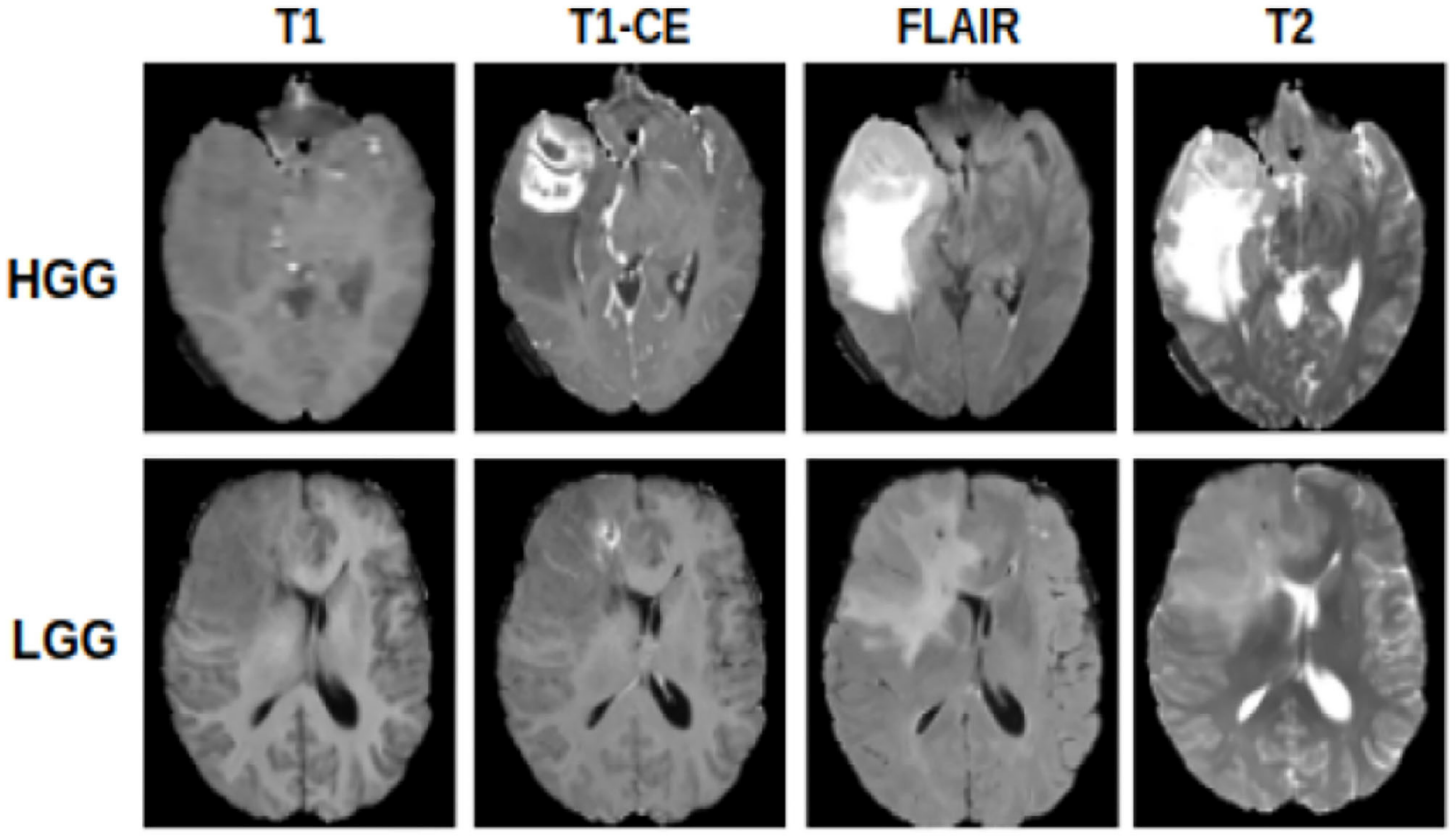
Examples of MRI slices of LGG and HGG samples. Each sample has four MR modalities, i.e., T1-weighted MRI, T1ce MRI, FLAIR MRI, and T2-weighted MRI.
